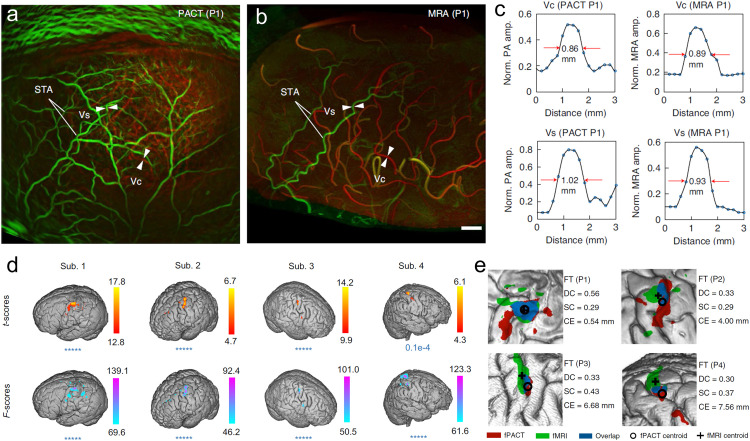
Massively parallel functional photoacoustic computed tomography of the human brain[76]. (a) Photoacoustic computed tomography angiography of human brain. (b) Magnetic resonance angiography of the same brain. (c) The diameters of the selected scalp vessel (Vs) and cortical vessel (Vc) of participant 1 were quantified as the full width at half maximum (red arrows). (d) Functional responses to sequential finger tapping were recorded in participants 1–4 by photoacoustic imaging. (e) The functional maps of sequential finger tapping.
